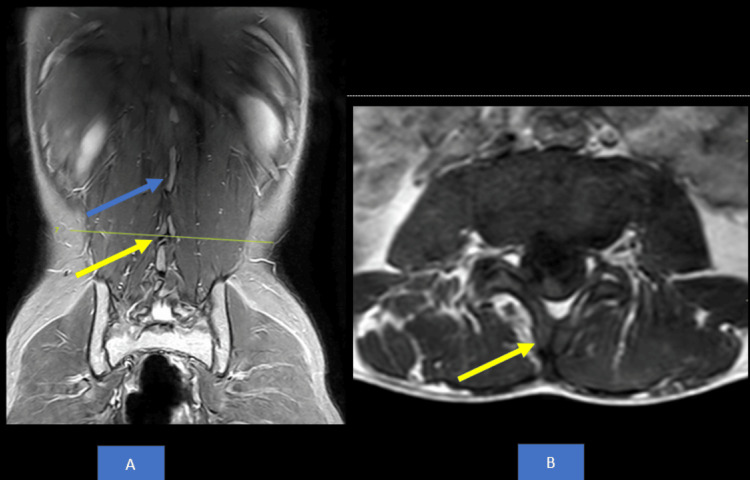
MRI of lumbosacral spine A) STIR coronal section, B) T1-weighted axial section, yellow arrow showing bifid L3 spinous process, blue arrow showing fusion of spinous process of L1 and L2 vertebra STIR: short tau inversion recovery
